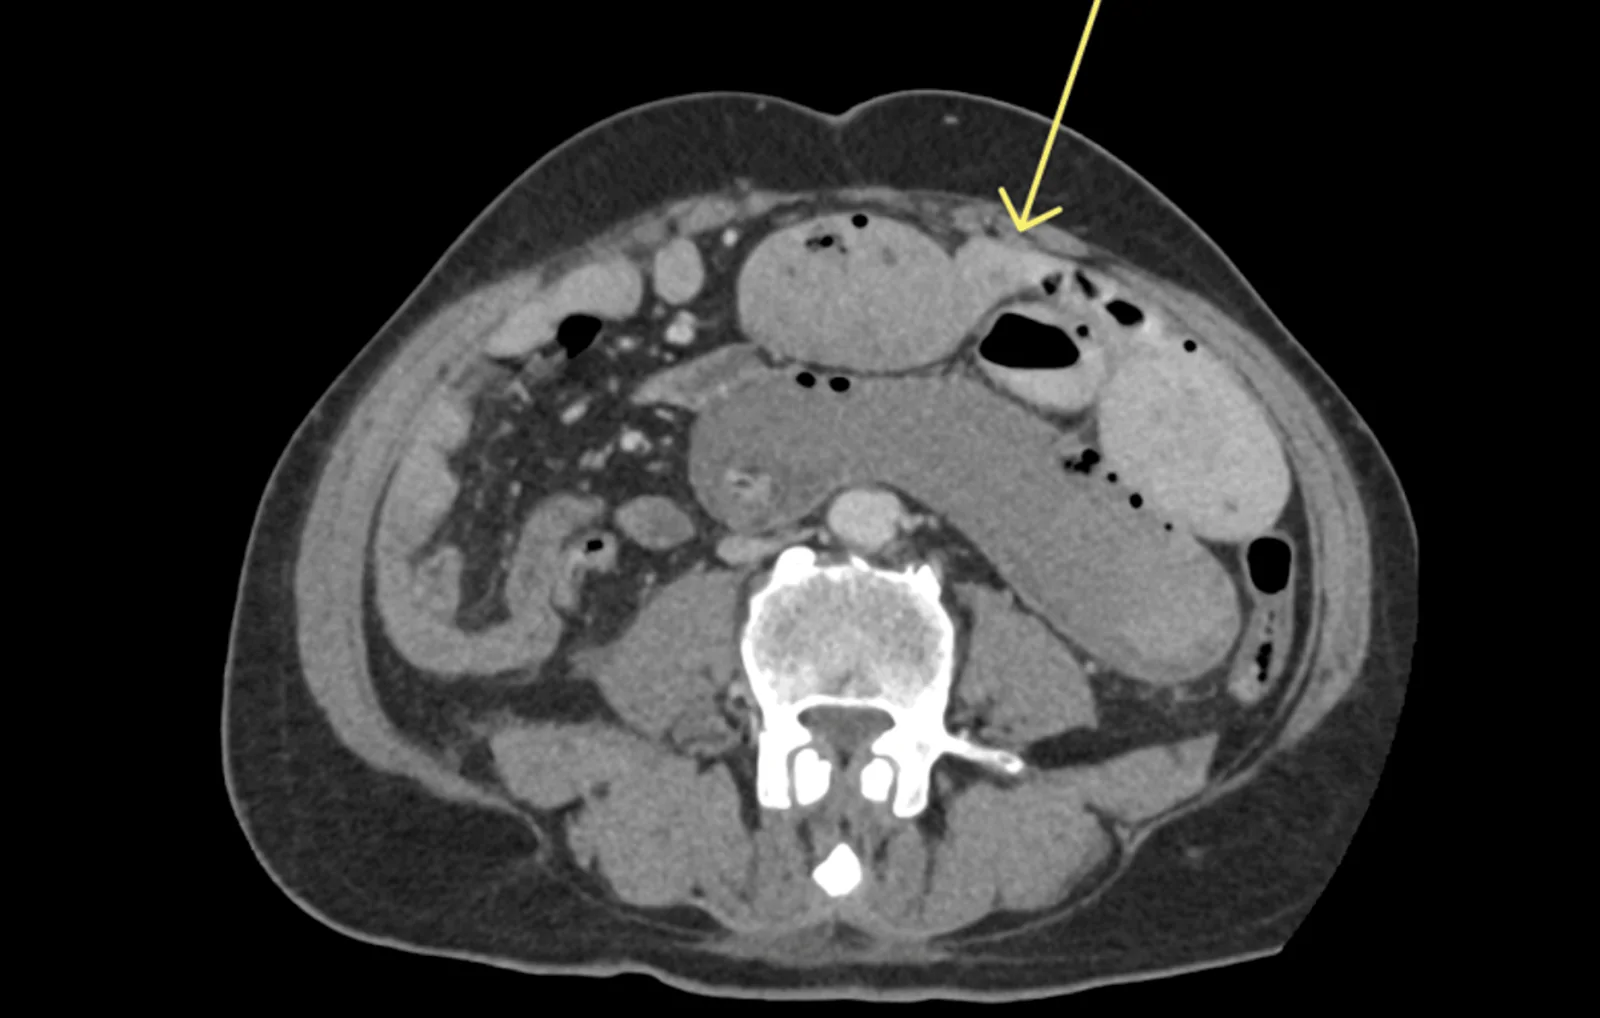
Axial contrast-enhanced abdominal CT performed approximately 72 hours after symptom onset demonstrating the abrupt caliber transition of the jejunal loop adjacent to the anterior abdominal wall (yellow arrow), corresponding to the site of mechanical obstruction.
